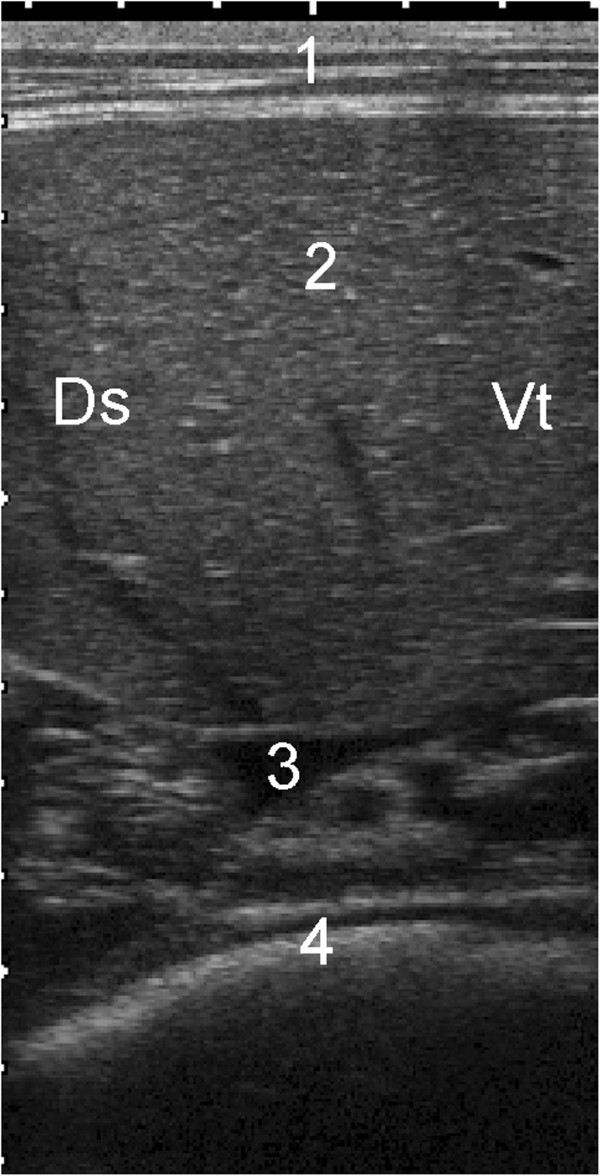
Ultrasonogram of the liver and caudal vena cava. Ultrasonogram of the liver and caudal vena cava viewed from the 11th intercostal space on the right in a 95-day-old Holstein-Friesian calf. 1 Abdominal wall, 2 Liver, 3 Caudal vena cava, 4 Rumen, Ds Dorsal, Vt Ventral. (depth of the image = 12 cm).
